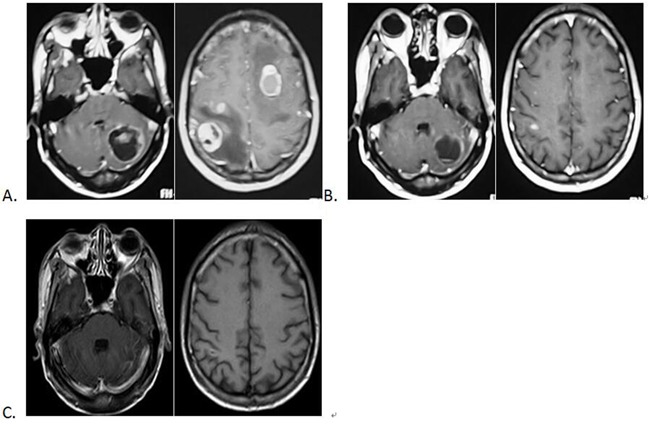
MRI images showing brain metastases of NSCLC A. Before the SRS treatment; B. One month after SRS treatment; C. Four months after SRS treatment.
